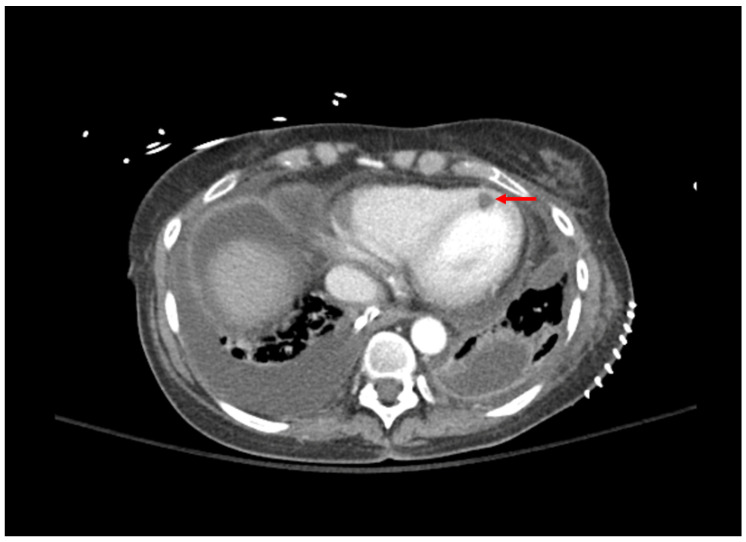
Computed tomography of the chest; bilateral pleural effusions grew *Aspergillus fumigatus*. Area of myocardial hypoperfusion consistent with fungal myocarditis is demonstrated by red arrow.
